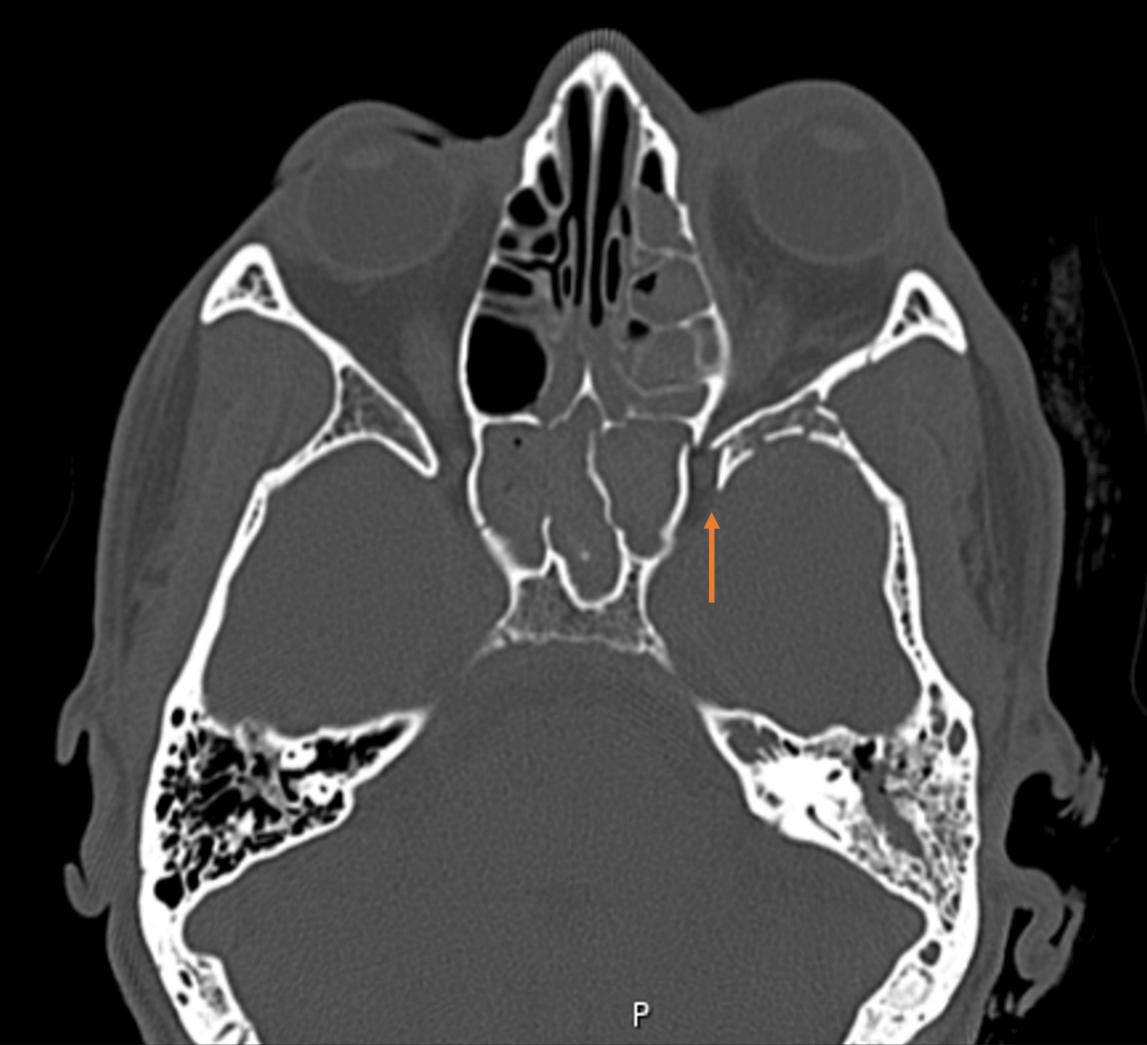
Preoperative CT imaging (axial view). The orange arrow highlights the preoperative narrowing of the superior orbital fissure caused by displaced bony fragments from the zygomaticomaxillary complex (ZMC) fracture.
